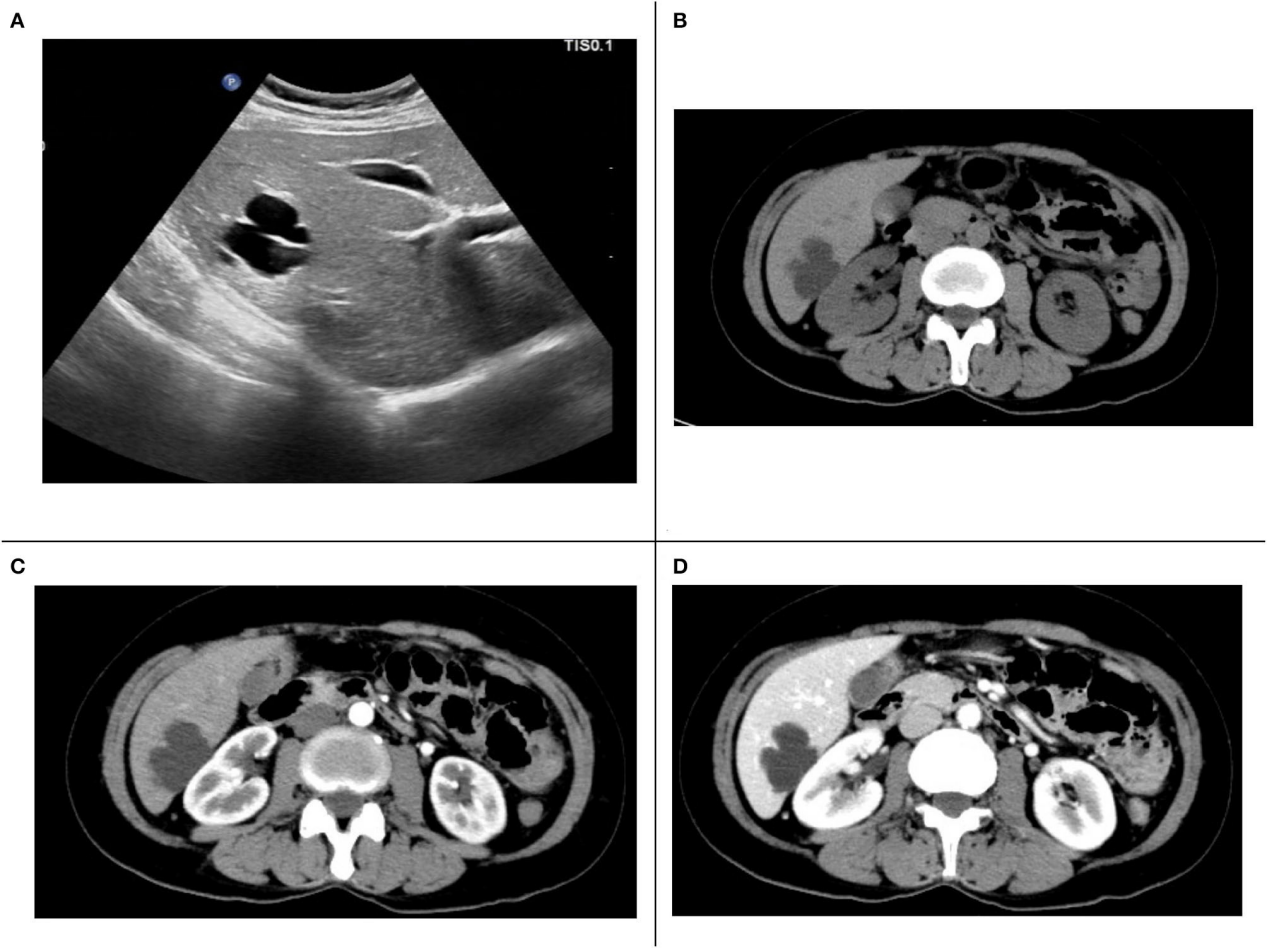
(A) Liver ultrasound. (B) Computed tomography (CT) plain scan. (C) The arterial phase of enhanced CT scans. (D) The portal phase of enhanced CT scans.
